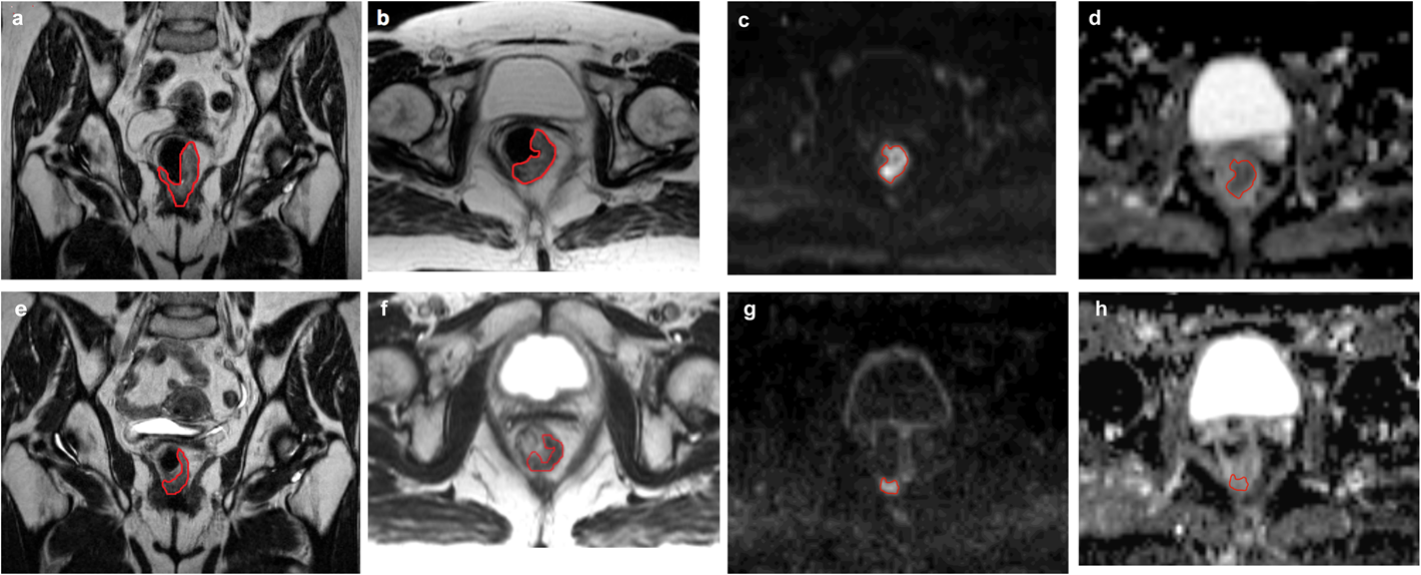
Example of tumor regression in a single patient between the first and last fraction of neoadjuvant treatment. Distinct decrease in gross tumor volume (GTV; red outlined) is evident (a + b: coronal and transverse T2-weighted images at baseline, e + f: coronal and transverse T2-weighted images at the end of neoadjuvant RCT). The diffusion-restricted area within the GTV (red) also declines until end of RCT, indicating the treatment-related cell depletion (c + d: transverse DWI sequence obtained with a b-value of 1000 s/mm^2^ and corresponding ADC-map at baseline, g + h: transverse DWI sequence (b-value = 1000) and ADC-map at the end of neoadjuvant RCT; the segmented GTV (red) on the ADC-maps is given to highlight the tumor, however segmentation for the ADC calculation has been performed on high b-value images
